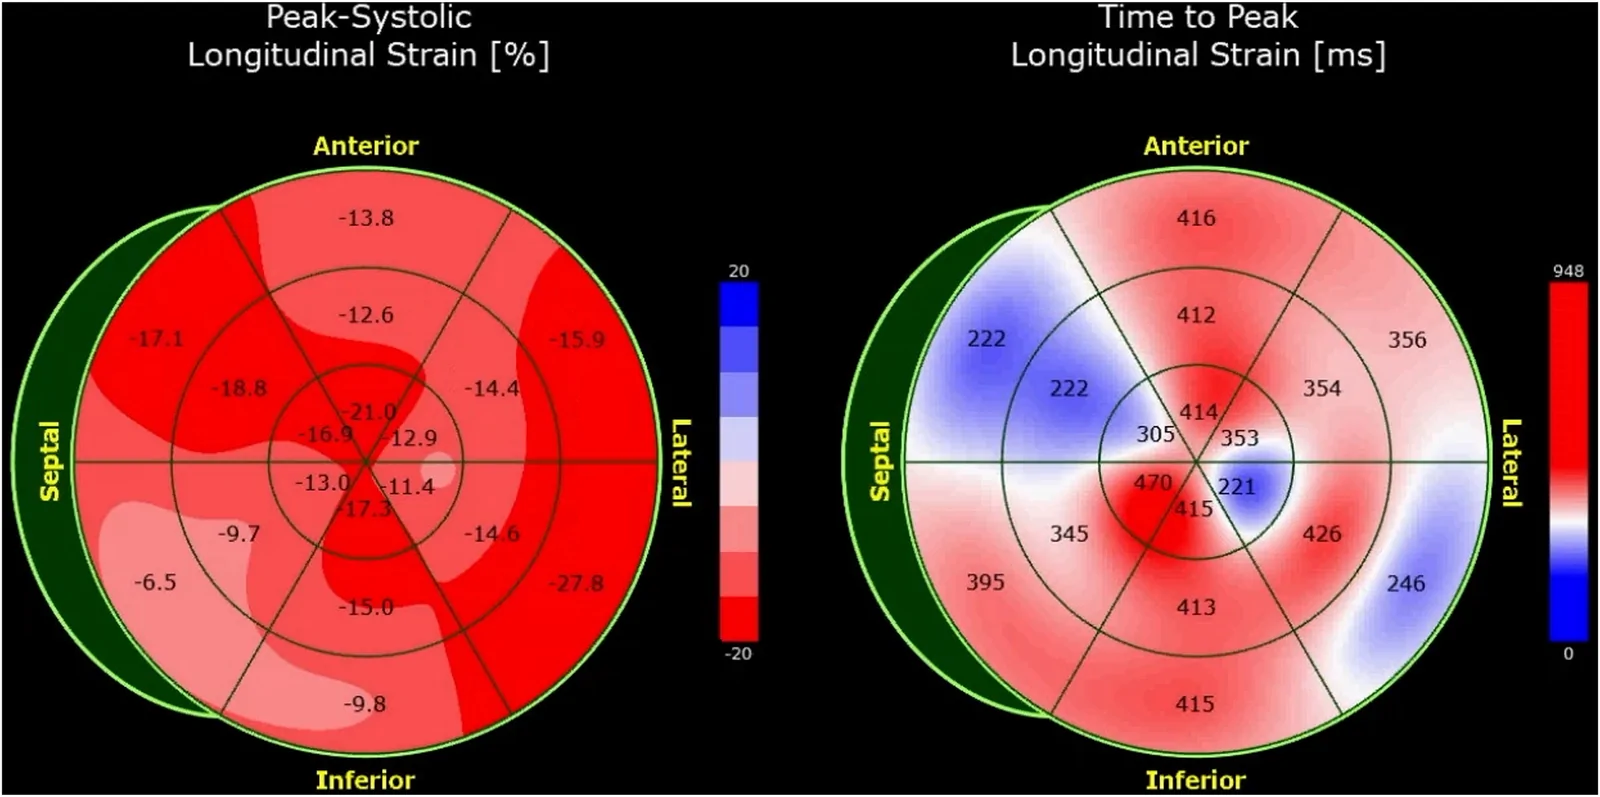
Echocardiogram With Strain Mildly reduced global longitudinal strain of −16.6%.
